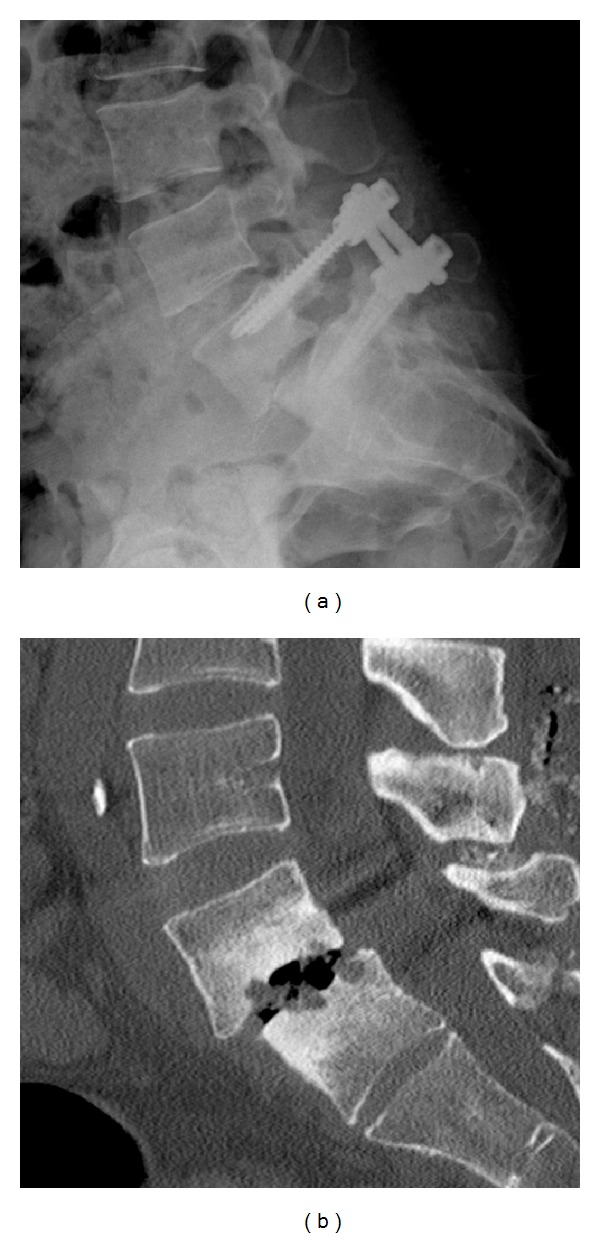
Lateral postop radiography (a) and postop CT scan (b) scan showed material in place, air in L5-S1 space, and reduction of the slippage L5-S1.
